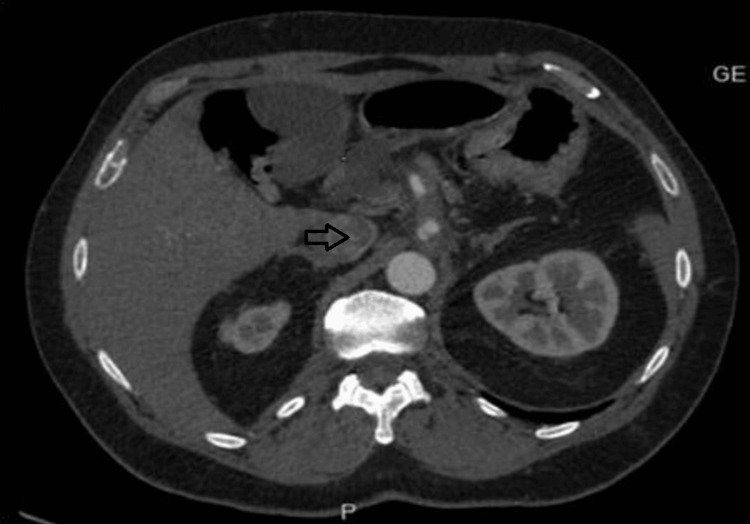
CT angiogram of the abdomen and pelvis showing a saccular pseudo-aneurysm (arrow)
